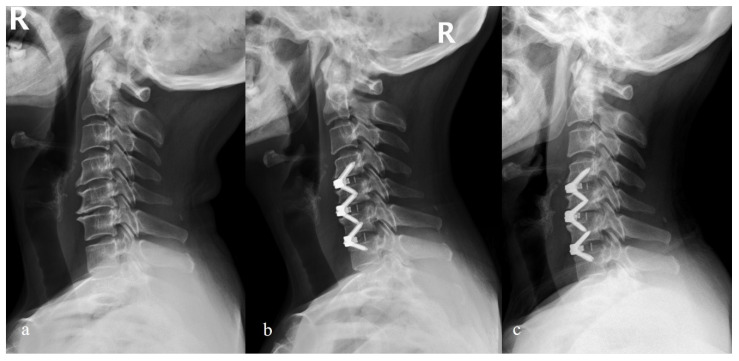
Lateral radiographs of one patient receiving Zero-P spacer system. (a) Preoperative C2-C7 Cobb angle was 5 degrees; (b) postoperative C2-C7 Cobb angle decreased to -5 degrees (kyphotic); (c) the C2-C7 Cobb angle was 0 degrees at the 2-year follow-up.
